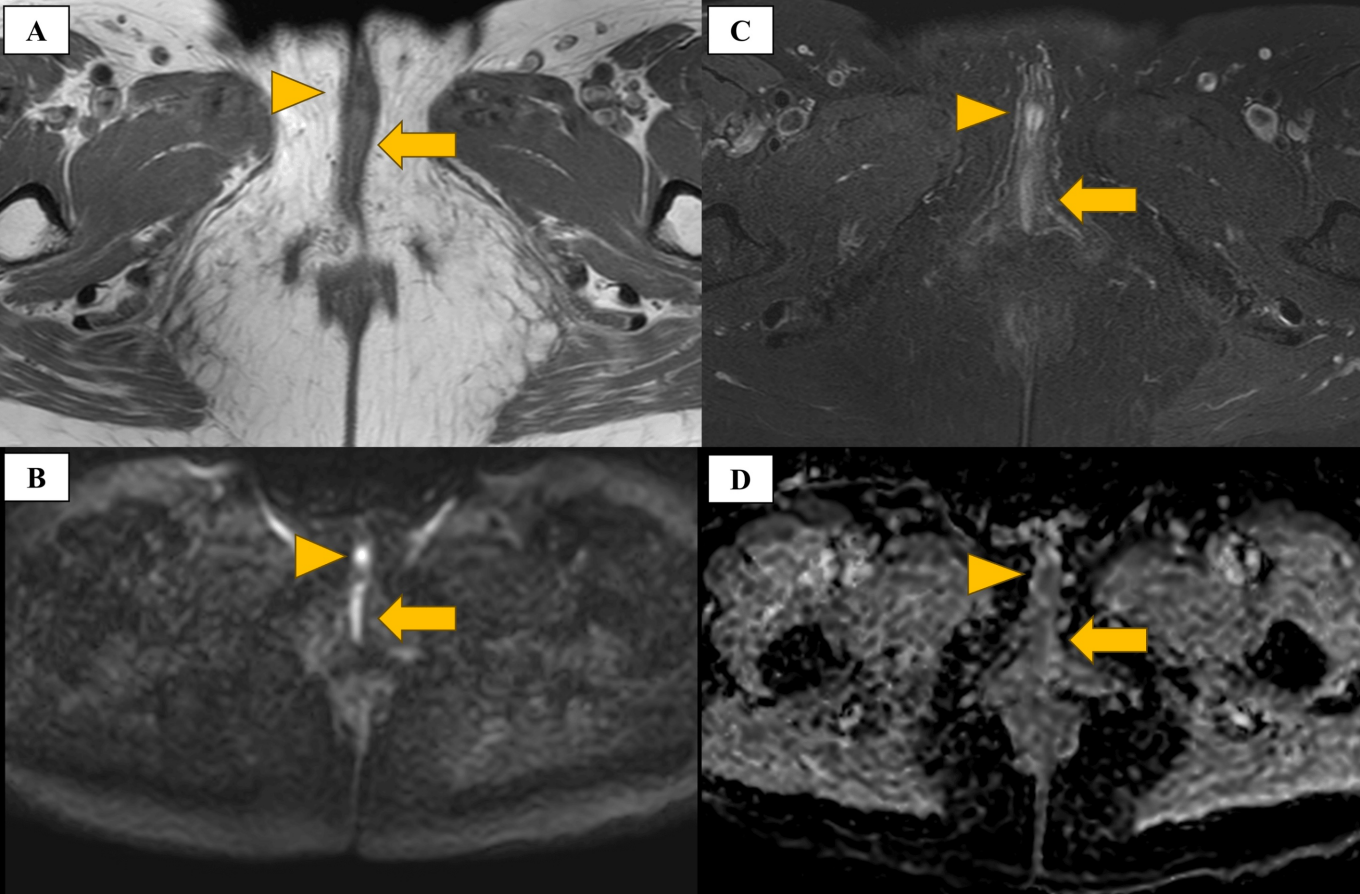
Pre-treatment MRI (A) T1-weighted image showing mild-high signal intensity in the vaginal wall (arrow) and urethra (arrowhead). (B) T2 fat-suppressed image showing high signal intensity in the vaginal wall (arrow) and urethra (arrowhead). (C) Diffusion-weighted image (b = 1000) showing high signal intensity in the vaginal wall (arrow) and urethra (arrowhead). (D) Apparent diffusion coefficient map showing a region of low signal intensity corresponding to a high signal intensity lesion on the diffusion-weighted image. MRI: magnetic resonance imaging
